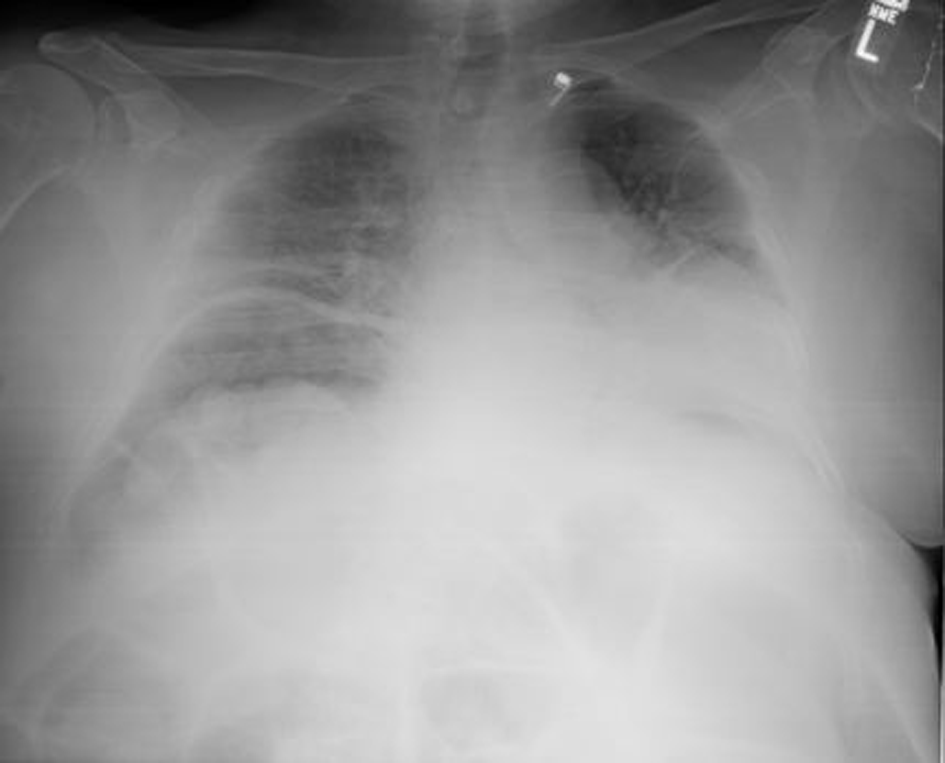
Chest X-Ray demonstrating colonic dilatation with absence of air under diaphragm.
